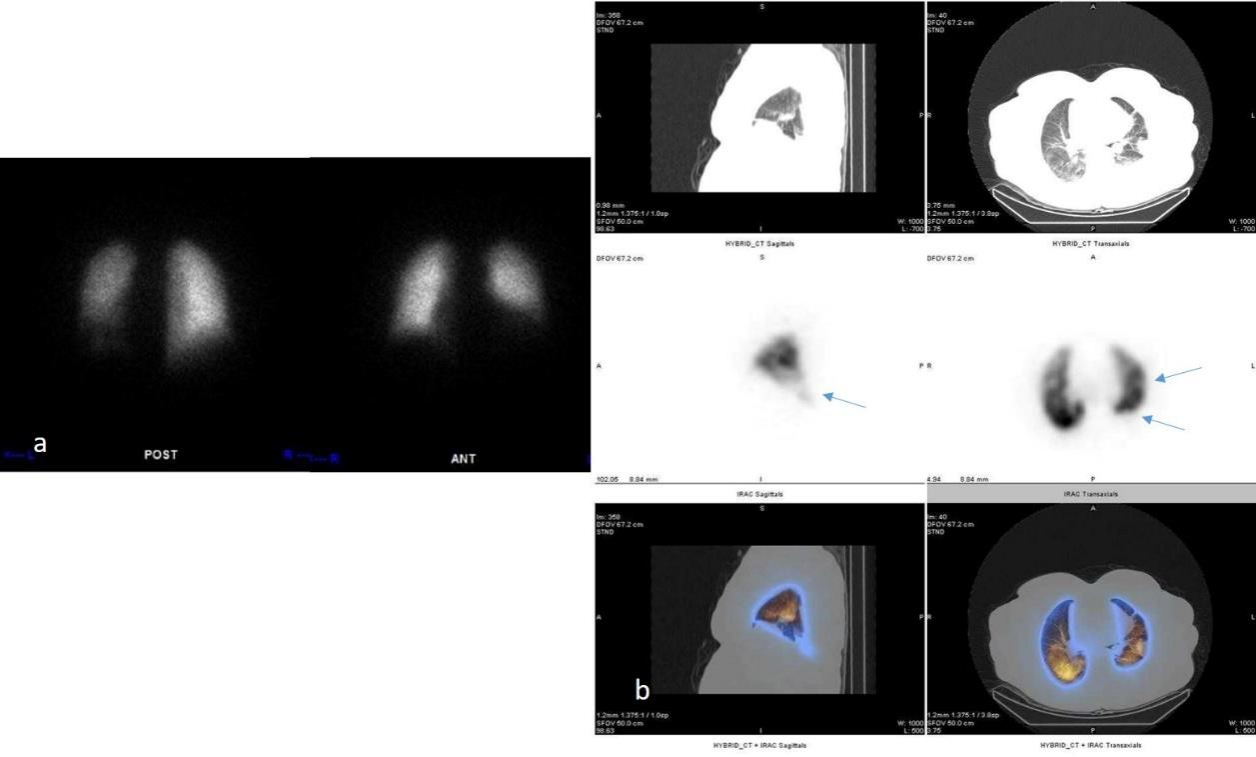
Lung planar (a) and SPECT/CT (b) perfusion scan of a patient. Perfusion defects in the left lung correspond to CT abnormalities, including consolidations and pleural effusion. The ventilation scan was not done. The scan was not compatible with PE. Blue arrows indicate the observed defects or abnormalities in the images
